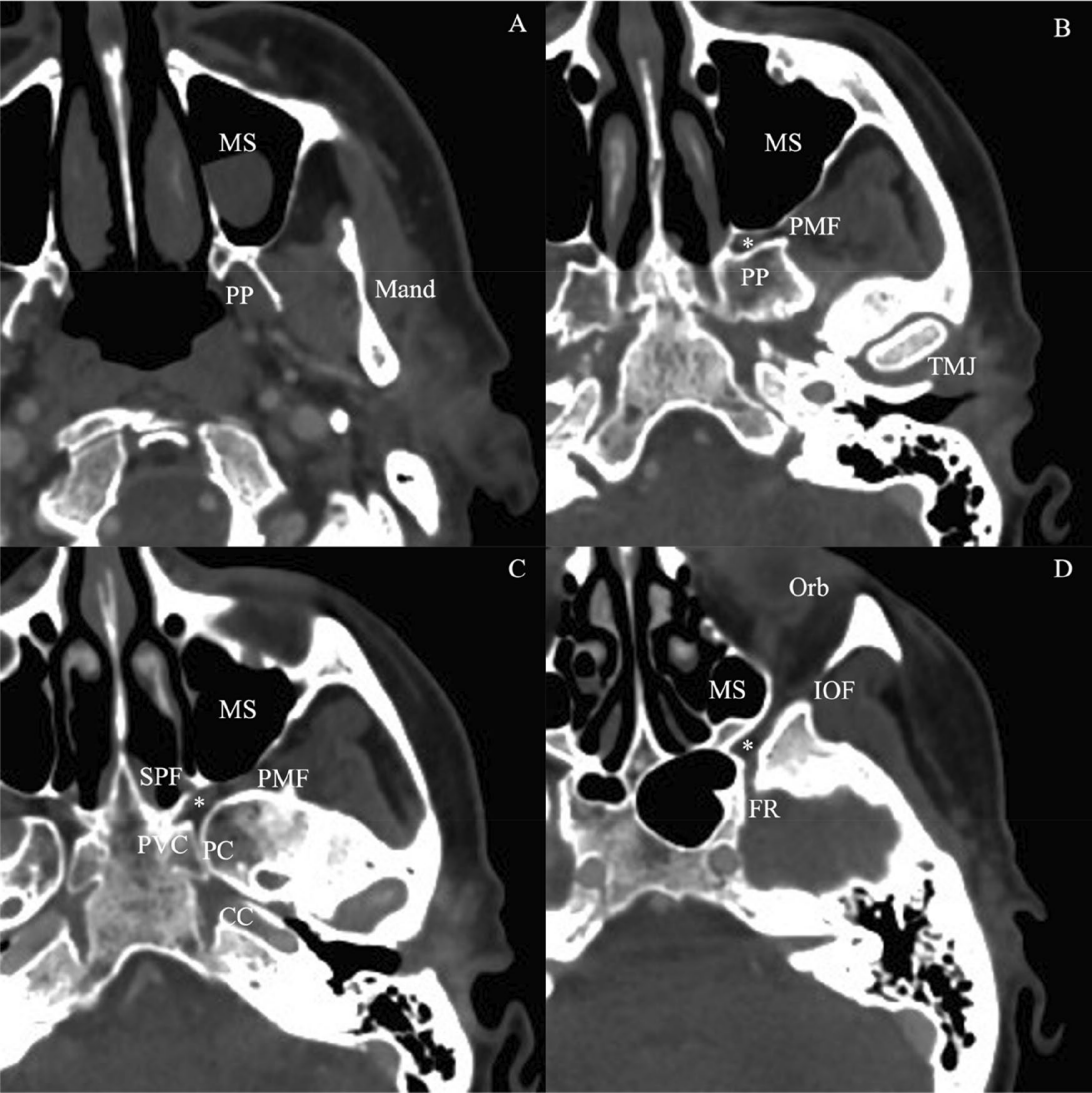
Radiologic anatomy of the pterygopalatine fossa at different levels on axial CT records. (A) Immediately above the hard palate, (B) at the level of the temporomandibular joint, (C) at the level of the carotid canal (petrous segment), (D) at the level of the orbit. (WW 1300 ± 80 HU; WL 500 ± 80 HU). *CC* carotid canal, *FR* foramen rotundum, *HU* Hounsfield unit, *IOF* inferior orbital fissure, *Mand* mandibula, *MS* maxillary sinus, *Orb* orbit, *PC* pterygoid canal, *PMF* pterygomaxillary fissure, *PP* pterygoid process, *PVC* palatovaginal canal, *TMJ* temporomandibular joint; *pterygopalatine fossa.
